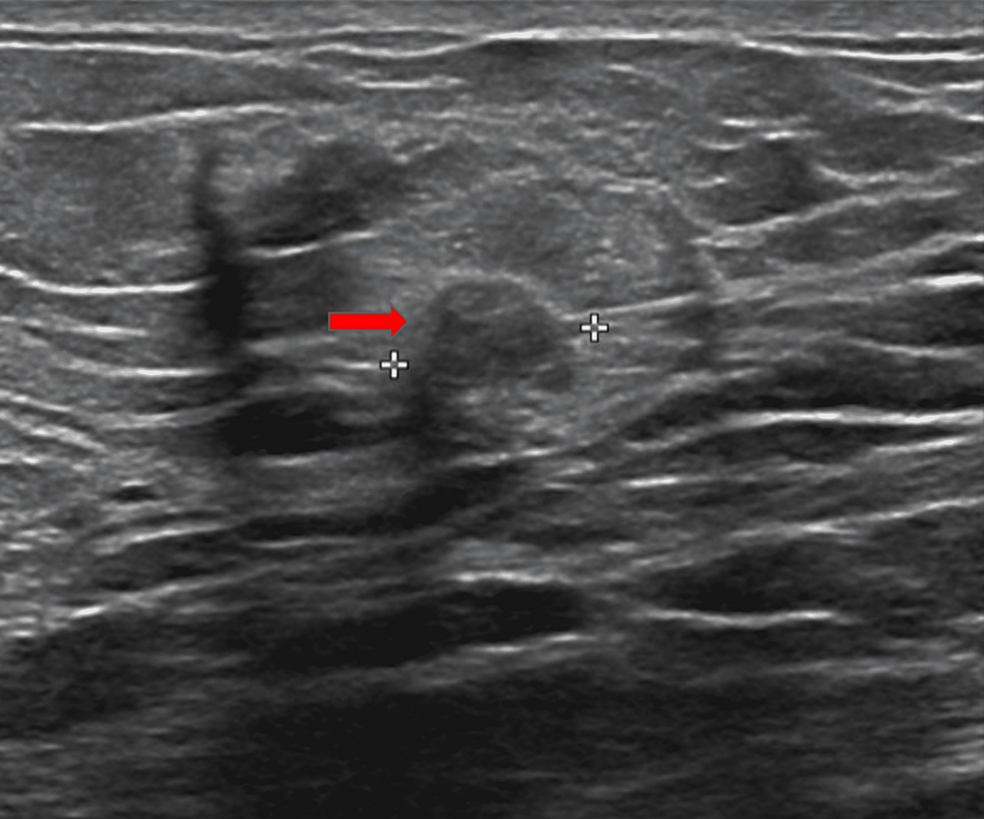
Ultrasound of right breast showing subareolar hypoechoic mass measuring 0.8 x 0.7 x 0.8 centimeter.
